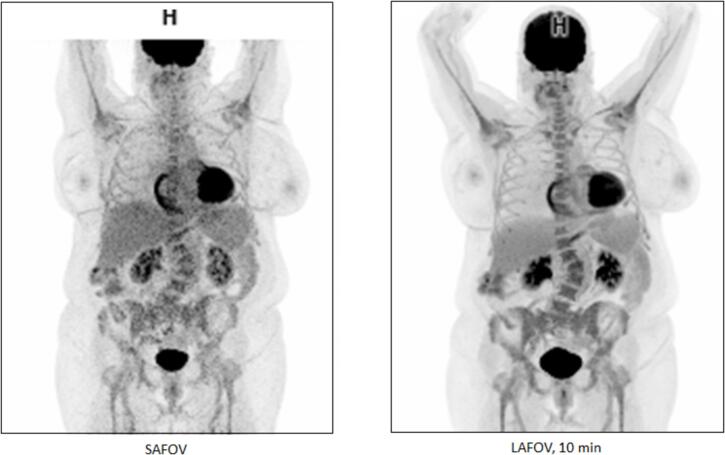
[^18^F]FDG PET maximum intensity projection images from (a) a short axial field of view scanner (264 MBq (3 MBq/kg), 15-minute acquisition), LAFOV (106 cm) with data acquisition for (b) 10 mins in a 70-year-old female patient with body mass index 37 kg/m^2^ demonstrating improved images quality and signal to noise in the LAFOV image.
